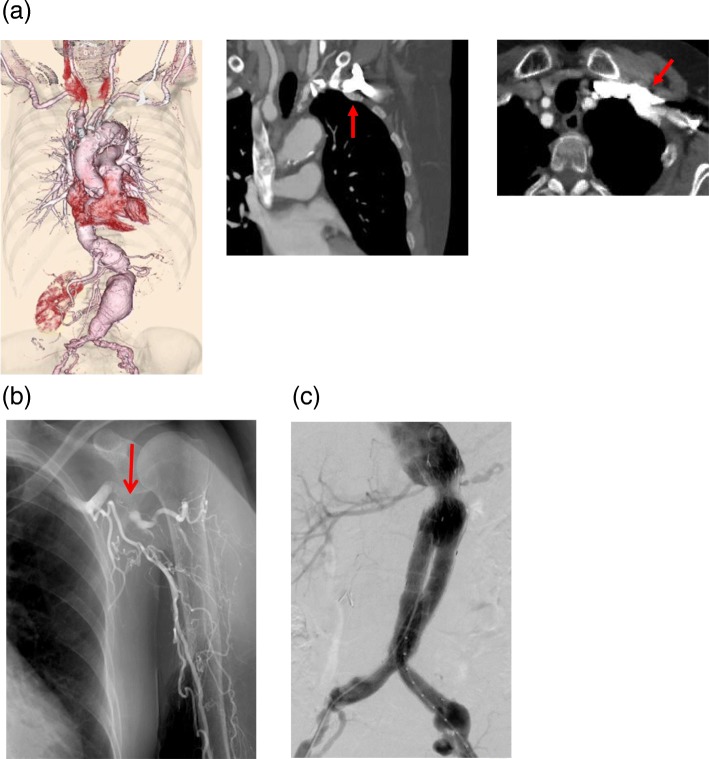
a In preoperative enhanced CT scan, AAO was not seen but was well calcified. Contrast is injected from the left upper extremity, and this causes severe halation at the axillar/subclavian vein, which makes visualization of the adjacent left subclavian artery difficult or impossible. b AAO was encountered and visualized during operation (arrow). The snorkel technique was abandoned, a regular EVAR was attempted, and accessing the right upper extremity was preserved as a fallback option following failed EVAR. c There were no endoleaks on completion angiography despite not performing the snorkel technique
